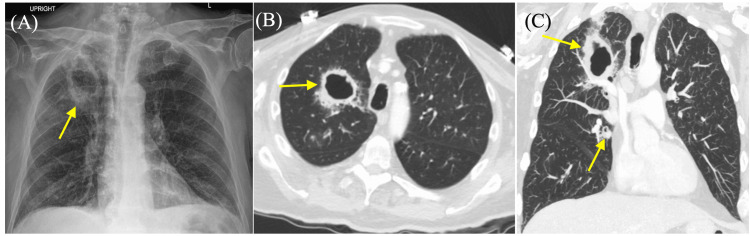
Radiography and computed tomography of the thorax (A) AP chest X-ray showing a cavitary lesion in the right upper lobe measuring ~6 cm. (B) CT chest (coronal) showing multiple cavitating nodular consolidations within the right upper lobe, measuring up to 4.4 x 3.8 cm in the right suprahilar region, with adjacent ill-defined ground-glass opacity. (C) CT chest (axial) showing a large cavitary lesion in the right upper lobe with surrounding inflammatory changes. AP, anteroposterior; CT, computed tomography.
